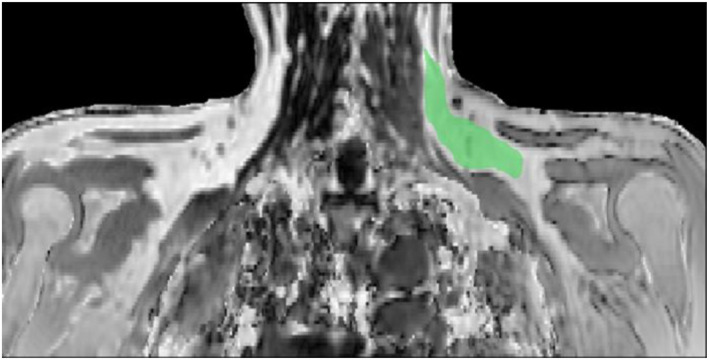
Example magnetic resonance image with left (postcooling) supraclavicular brown fat depot region of interest highlighted green. Images taken pre‐ and post‐60 min seated cooling and 14‐day beetroot intervention.
